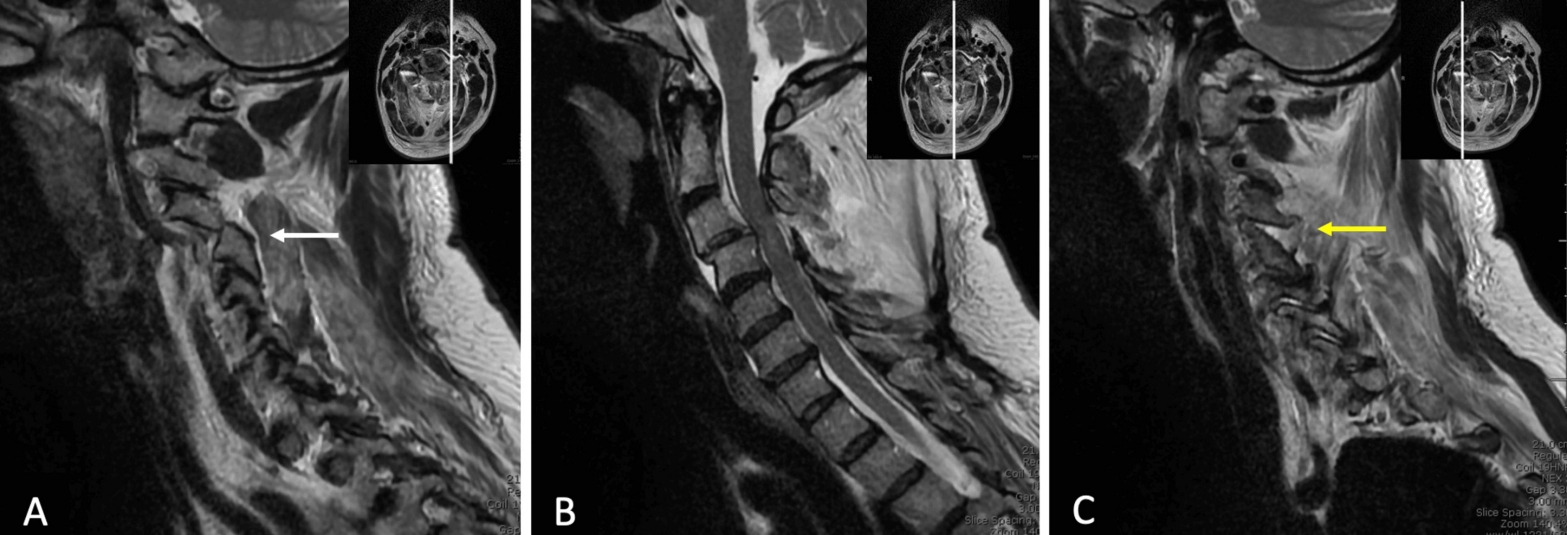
(A,B,C) Pre-operative magnetic resonance imaging (MRI) of the cervical spine demonstrating a left unilateral C3-4 facet dislocation (white arrow). The right parasaggital image (C) demonstrates the distracted right C3-4 facet (yellow arrow).
